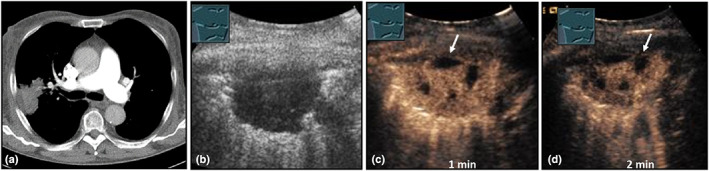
A 77‐year‐old patient presented with gastrointestinal stromal tumour under imatinib therapy, with refractory cough, bloody sputum, and known heart failure. Visualisation of a right‐sided, peripheral consolidation on computed tomography (courtesy of Prof. Dr. Andreas H. Mahnken, Department of Radiology, University Hospital Marburg) (a); B‐mode ultrasound shows a homogeneous, hypoechoic, smooth‐bordered consolidation (b); contrast‐enhanced ultrasound reveals an inhomogeneous perfusion with areas of absent perfusion (arrows) and mild washout of perfused areas after 1 min (c) and after 2 min (d). Ultrasound‐guided biopsy for suspected carcinoma revealed a diagnosis of organising pneumonia.
